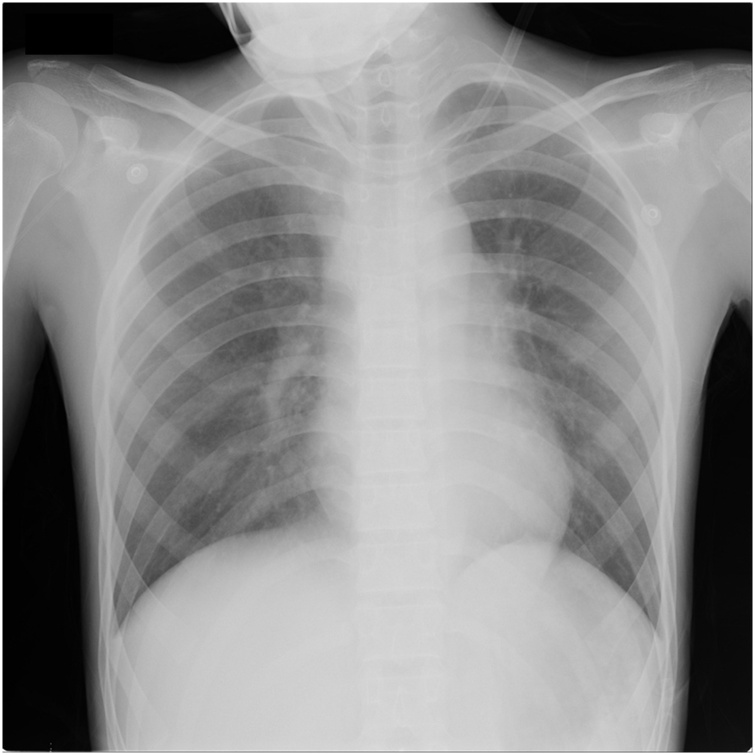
Chest X-ray three months post-chemotherapy showing tumor reduction.
